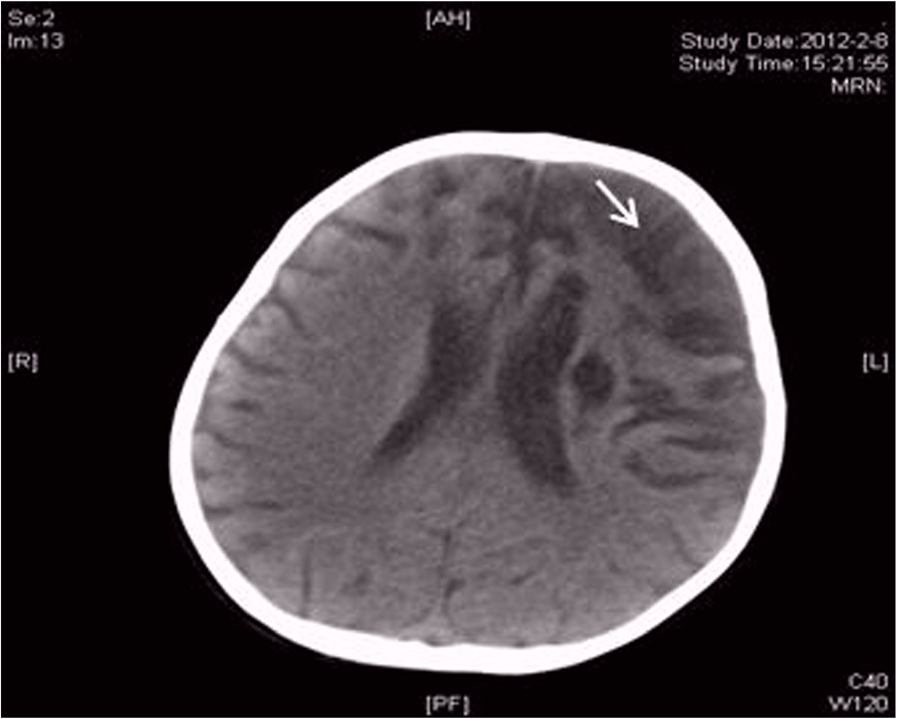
CT revealed low density imaging in the left parietal-temporal lobe, left basal ganglia and right frontal parietal-temporal lobe.
